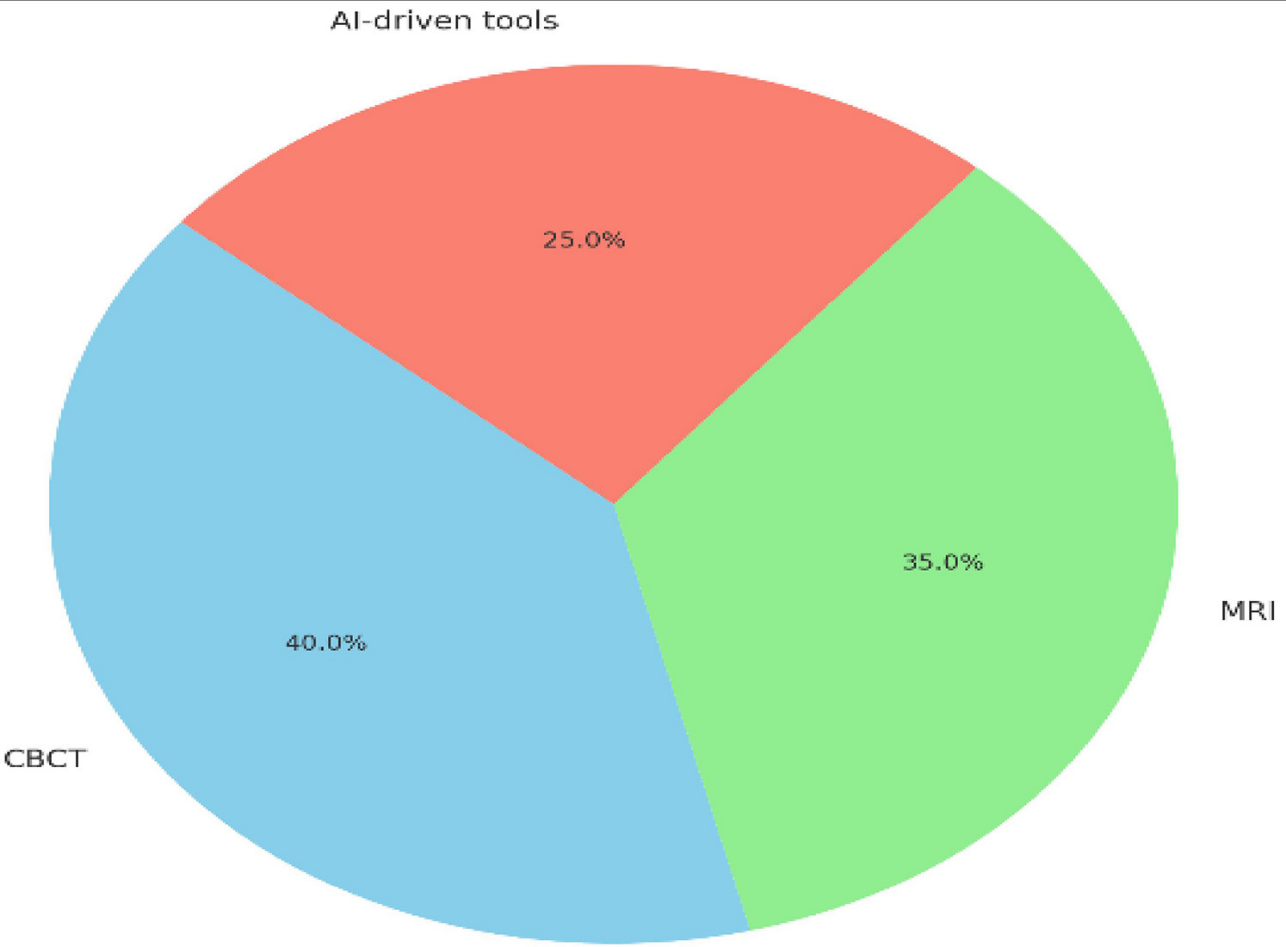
Pie chart showing the contribution of CBCT (40%), MRI (30%), and AI tools (30%) to innovations in precision dentistry.
